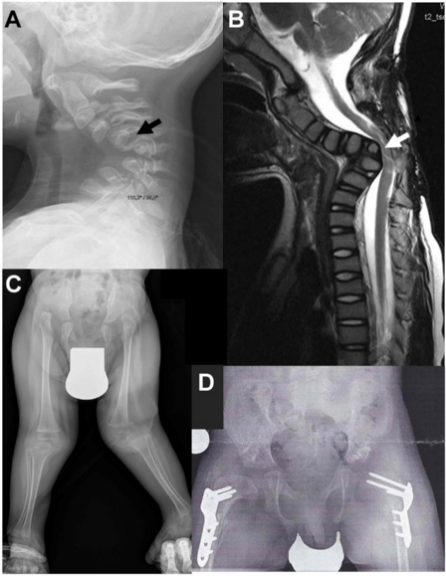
Radiographs of patient 7. Cervical lateral spine at 2 4/12 years: Cervical kyphosis of ~70° with dorsal cervical listhesis of vertebral bodies C4/C5 and C5/C6 (black arrow), dysplasia of vertebral body C6 (a). Cervical myelogram at 3 years: cord compression and myelopathy at C5/C6 (white arrow) (b). Radiograph of the hip and legs AP: note bilateral hip dysplasia with flat acetabulum, valgus position of femoral necks, luxation of both hip joints with cranial position of the femoral head and knee luxation on both sides (c). Radiograph of the hip AP: corrected position after operative hip replacement by acetabular reconstruction and femoral osteotomy (d). AP: anteroposterior
